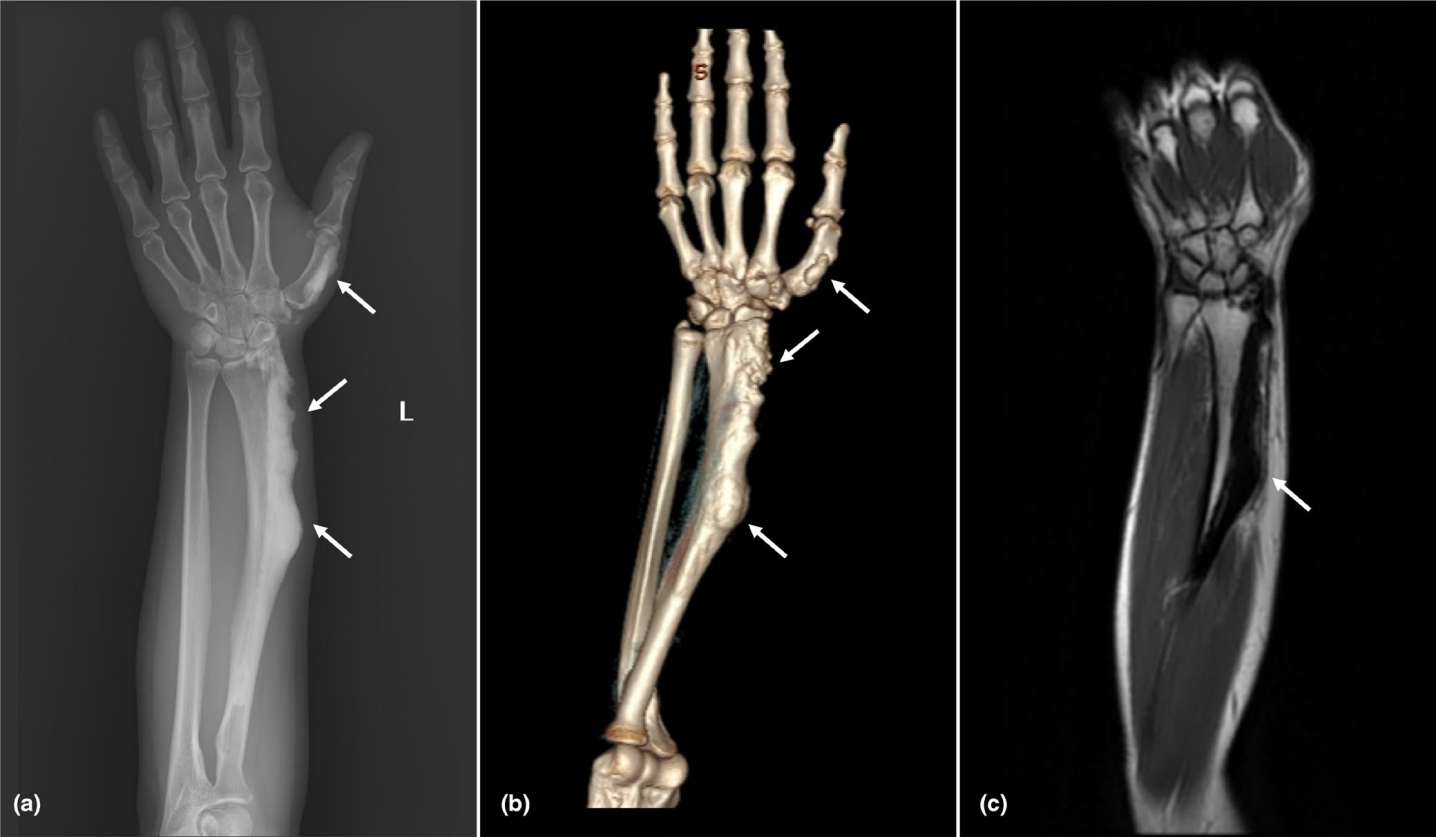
Multimodal imaging of melorheostotic lesions involving the left radius and first metacarpal. (a) Plain radiograph showing irregular, eccentric cortical thickening along the lateral aspect of the left radius and first metacarpal bone, with intramedullary extension. White arrows indicate the sites of cortical involvement. (b) Three‐dimensional reconstruction of the forearm and hand, demonstrating an eccentrically located extraosseous lesion arising from the cortex. White arrows highlight the abnormal cortical thickening. (c) Coronal T1‐weighted MRI showing a well‐circumscribed, eccentrically located lesion involving the distal two‐thirds of the left radius. The lesion appears hypointense and lacks soft tissue extension. The white arrow indicates the site of cortical involvement.
